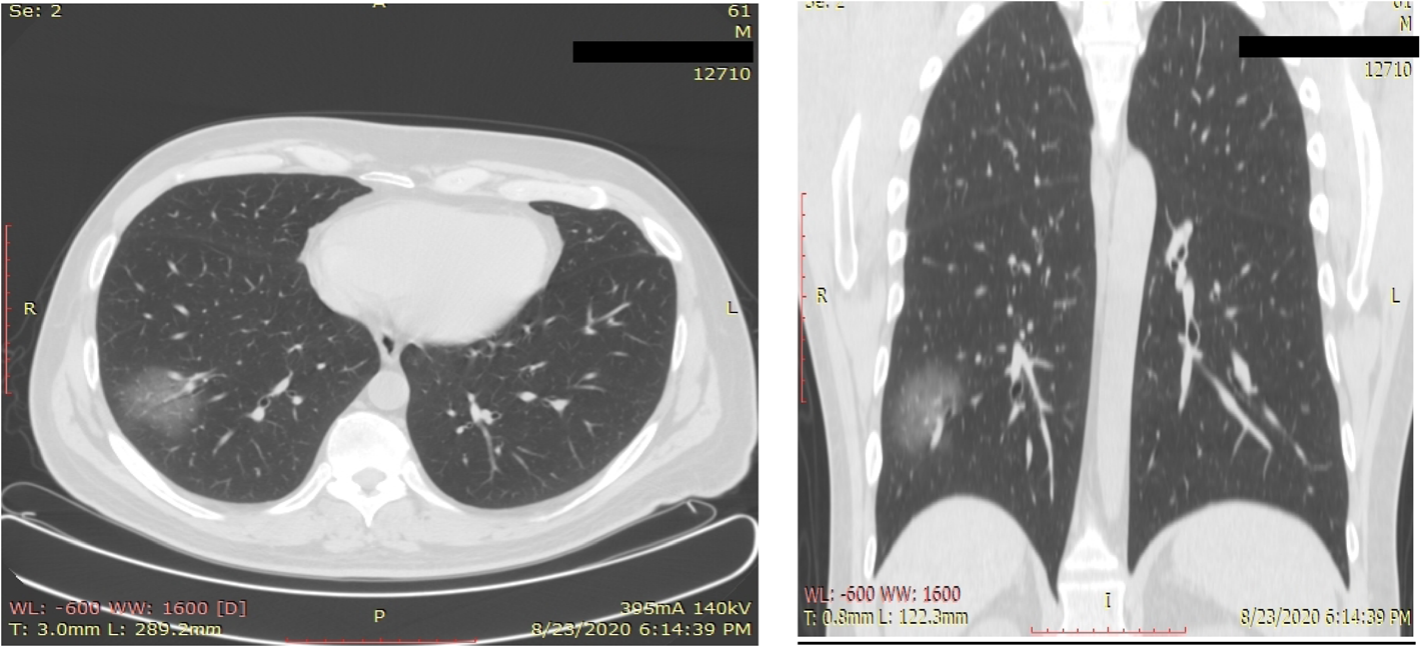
Thirty-two-year-old female patient presented with sore throat and mild fever. Her PCR test was negative, and the CT revealed unifocal ground glass opacity peripherally located in the right lower lobe. Lung involvement was less than 5%, i.e., CT severity score is 1
